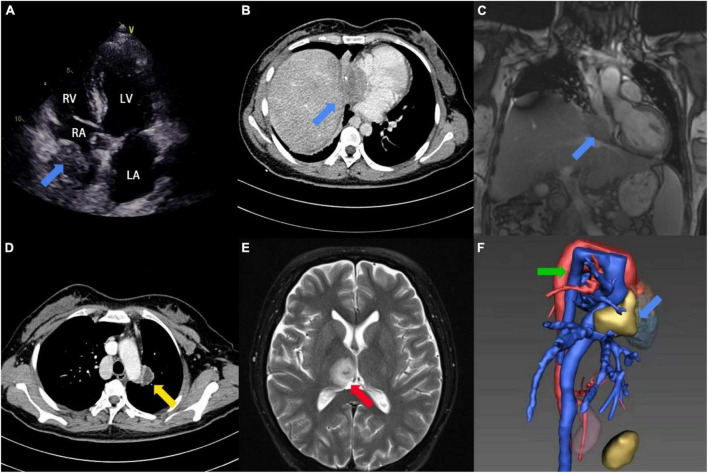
Image findings. (A) Preoperative 2D-TTE four-chamber view showed an irregular mass (blue arrow) within the right atrium. (B) Preoperative CTA showed a mass with slight calcification (blue arrow) within the right atrium and IVC. (C) Preoperative MRI showed a hypointense mass (blue arrow) at the junction of the right atrium and IVC on coronary-reconstruction view. (D) Preoperative CTA showed a lesion with slight enhancement in the left lung (yellow arrow). (E) Preoperative MRI showed an oval hypertense lesion (red arrow) within the brain on T2-weighted image. (F) 3D-reconstruction view of preoperative CTA showed the cardiac mass (blue arrow) and enlarged azygos vein (green arrow).
